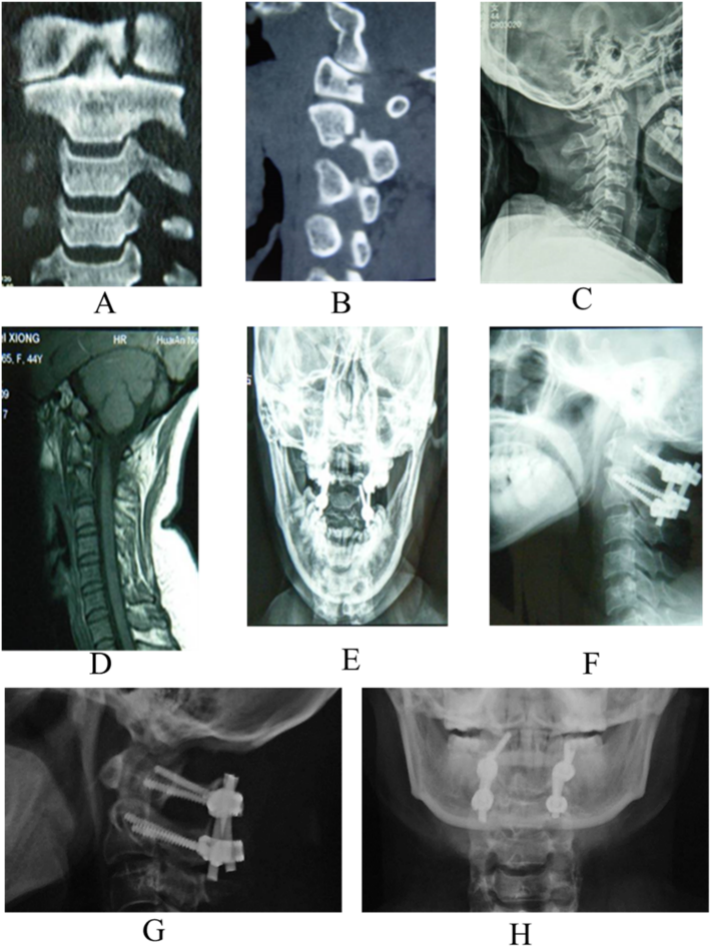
A 47-year-old male patient was admitted to our hospital due to neck pain and restricted neck motion for 2 h from falling from a height. Preoperative three-dimensional CT scanning (A), X-ray (B), and MRI (C) showed that anterior ring and hangman’s fracture. He underwent posterior atlantoaxial pedicle screw internal fixation. Postoperative anteroposterior (E) and lateral (F) X-ray showed that the atlantoaxial fracture was fixed well by the posterior atlantoaxial pedicle screw. At 9 months of follow-up, the anteroposterior (G) and lateral (H) X-ray showed that the fracture was healed well.
